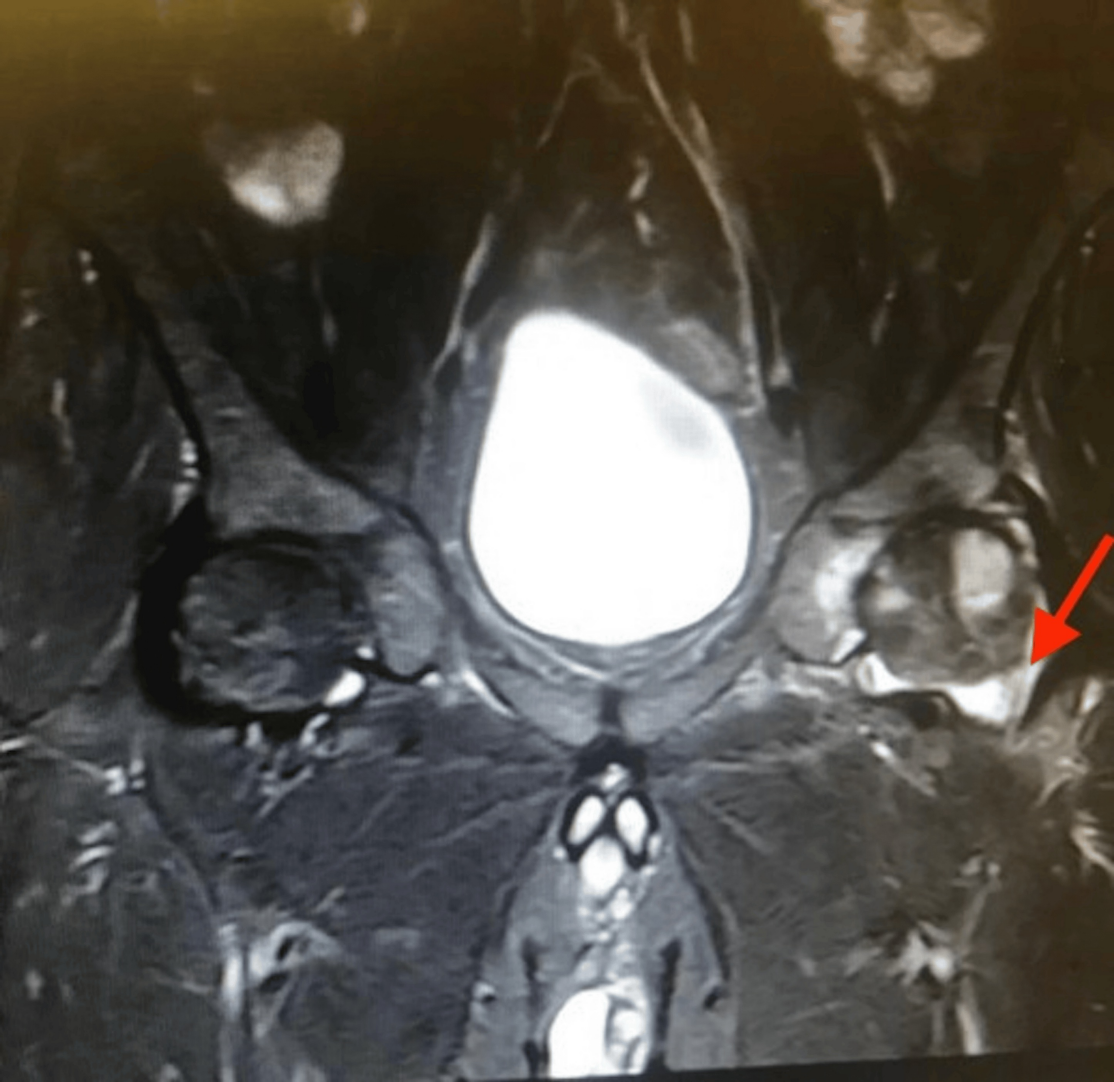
MRI showing left hip joint effusion Red arrow: effusion seen in the left hip joint MRI: magnetic resonance imaging
